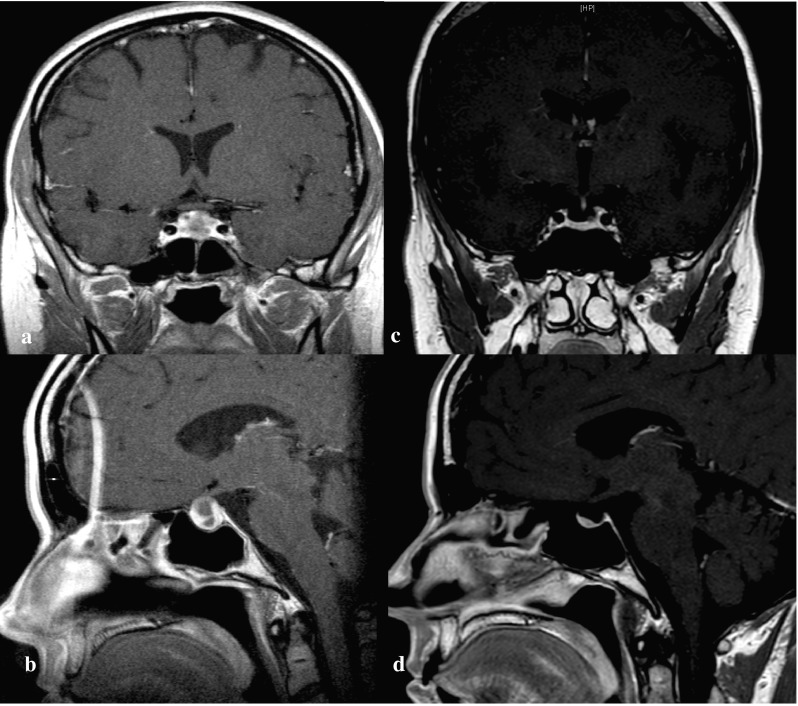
T1 weighted MRI (coronal and sagittal view) of a 37-year-old woman with GPA (Case 1) showing a sellar mass with peripheral enhancement and central cystic lesion at onset (a and b) and 8 years after treatment showing a partial empty sella (c and d)
